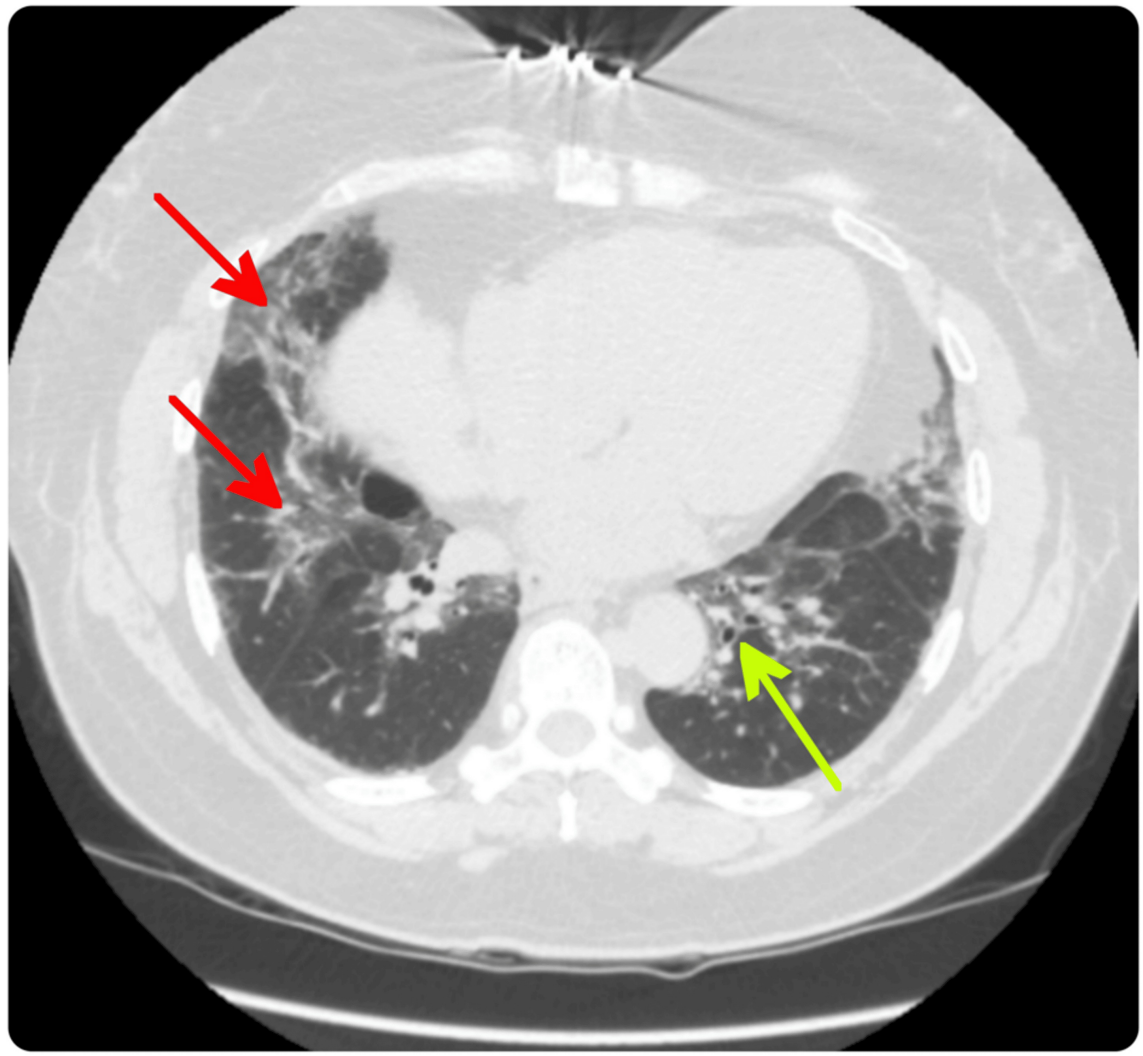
Follow-up HRCT in 2025 demonstrating bibasilar fibrotic scarring (red arrows) and mild bronchiectasis (green arrow), indicating a quiescent phase of connective tissue disease-associated ILD. HRCT: high-resolution computed tomography; ILD: interstitial lung disease
